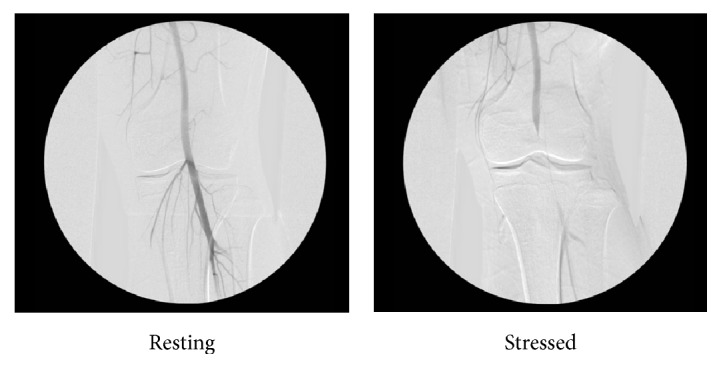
Stress CT angiography, left lower extremity. Notice near complete cessation of flow in the popliteal artery during the stressed or active phase of angiography.
